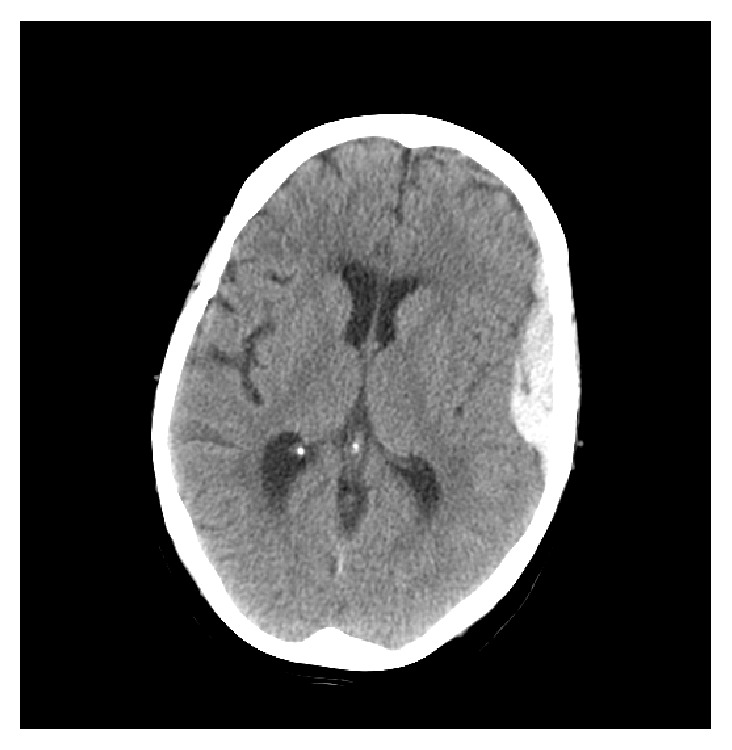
Repeat axial CT head without contrast.
